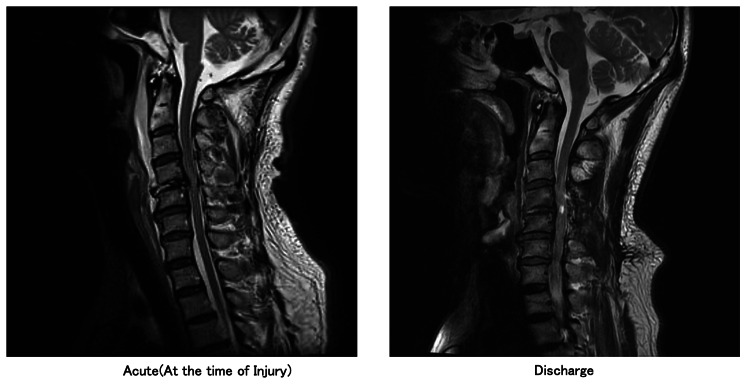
MRI images (Lt: acute, at the time of injury; Rt: discharge).
